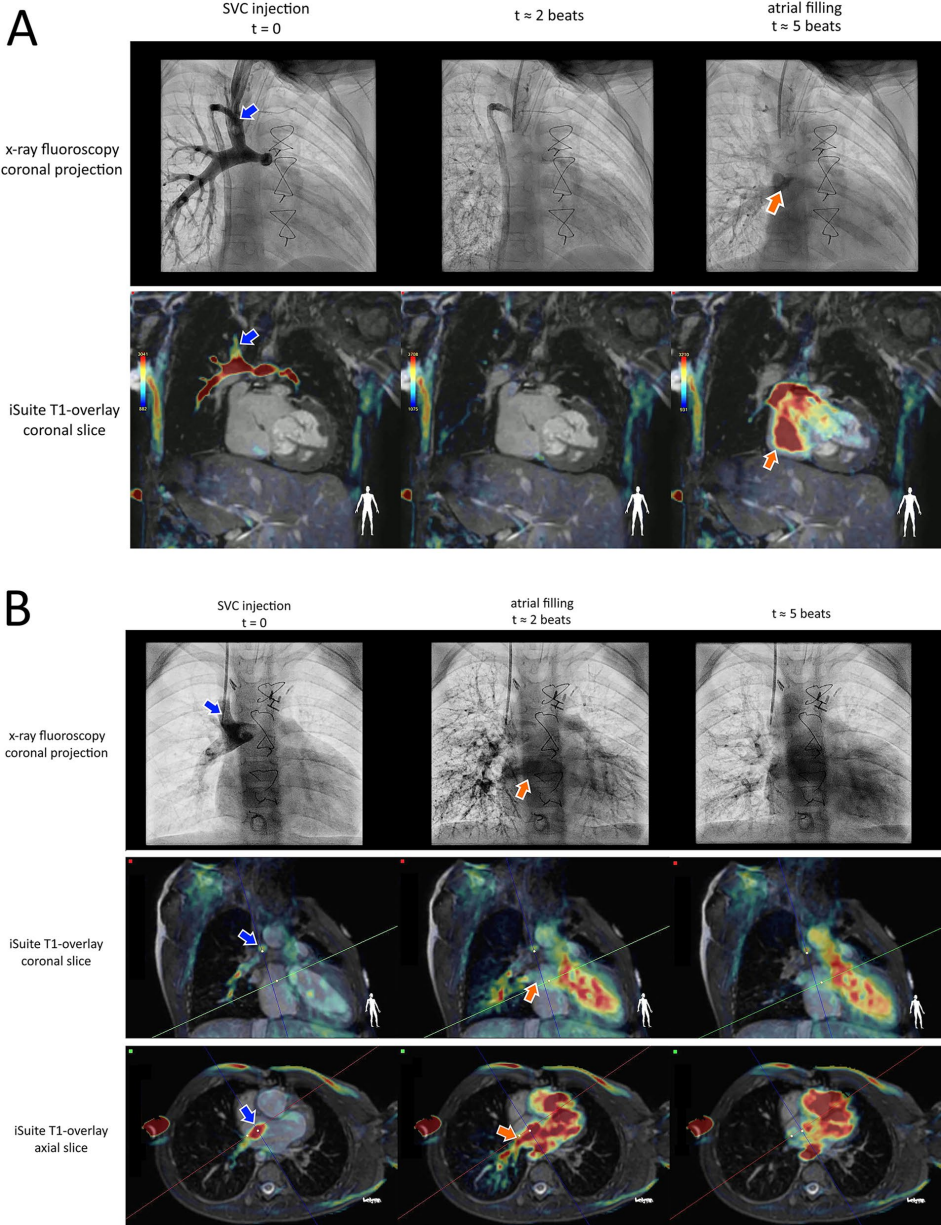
A Selective injection angiogram in a patient with a right sided Glenn with a normal pulmonary transit time. From left to right, the panels show the flow of the injected contrast agent from the superior vena cava (blue arrows), directly to the lungs, and back into the heart over several heartbeats with x-ray fluoroscopy and CMR in coronal planes. A manually-selected threshold was applied to the T1-weighted CMR to overlay the bright gadoliniumsignal on the high-resolution 3D dataset acquired at the beginning of the session, providing real-time visualization of the contrast flow patterns. In this patient (A), atrial filling (orange arrow) was not observed until the 5th heartbeat (third column) with both modalities, indicating normal transit time through the lungs. B Selective injection angiogram in another patient with a right sided Glenn (blue arrow) with T1-overlay images acquired in coronal and axial planes simultaneously. The second time point (middle column) shows rapid atrial filling (less than 2 heartbeats after the injection) with both modalities, suggesting the presence of pulmonary arteriovenous malformations (AVMs) in the right lung, which were confirmed by fluoroscopy in this patient. Videos of these selective angiograms can also be seen in Additional file 3
